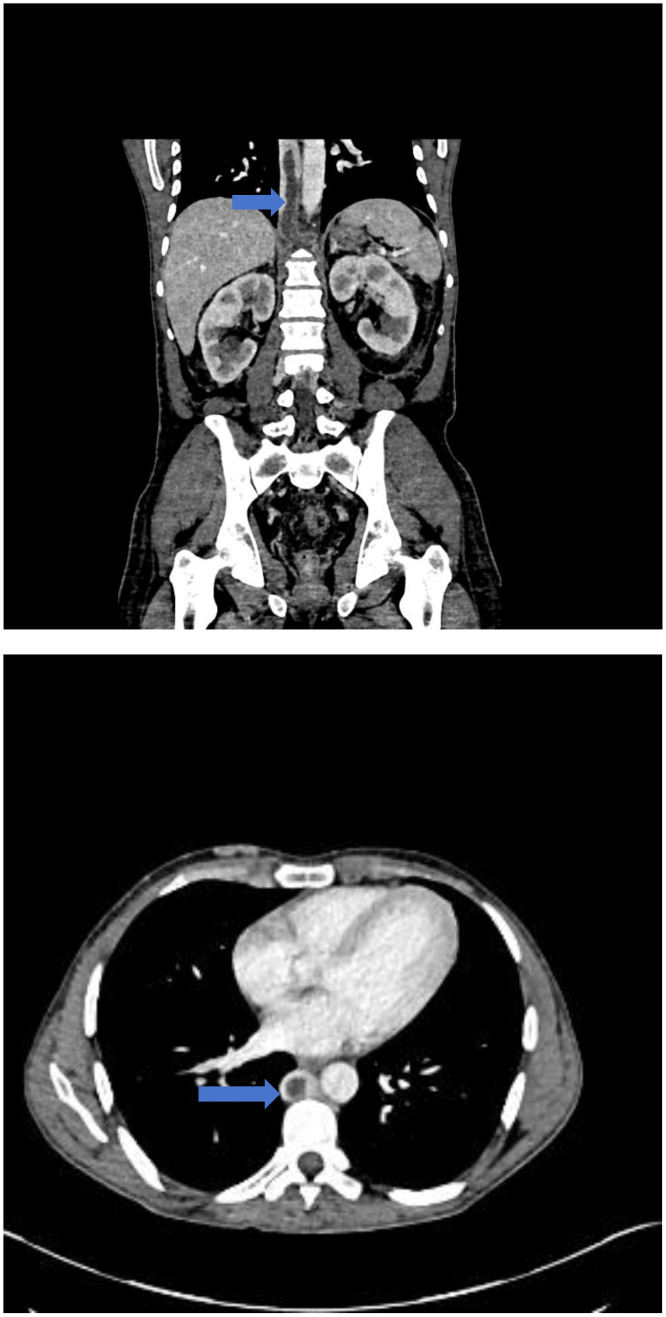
Abdomino-pelvic CT scan: Coronal and axial plans showing an enlarged azygos vein with thrombus (Blues arrows).
